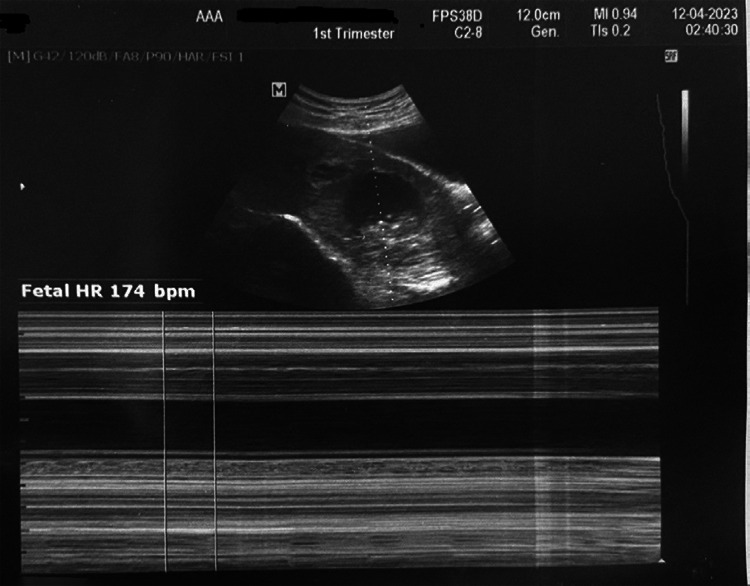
Ultrasonogram showing fetal cardiac activity.
